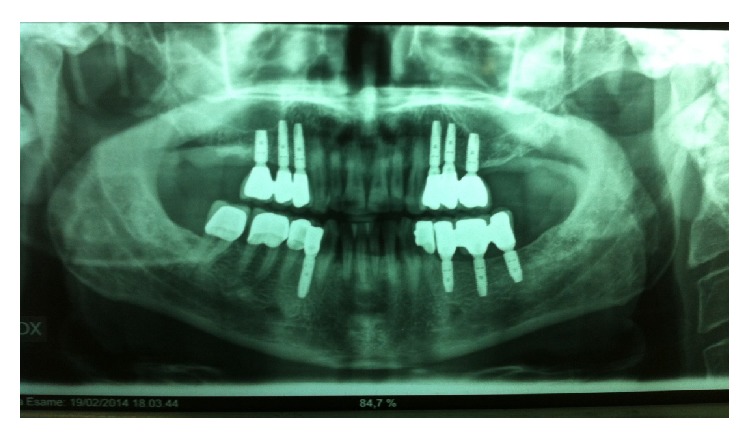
OPT radiographic situation 1 year after loading.
